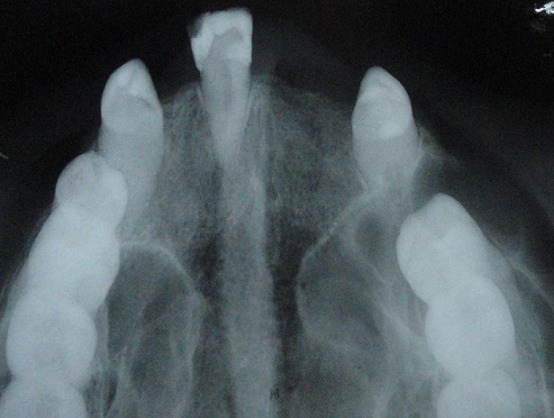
Maxillary true occlusal radiograph showing expansion of buccal cortical plate in region of 23, 24, 25 and 26
